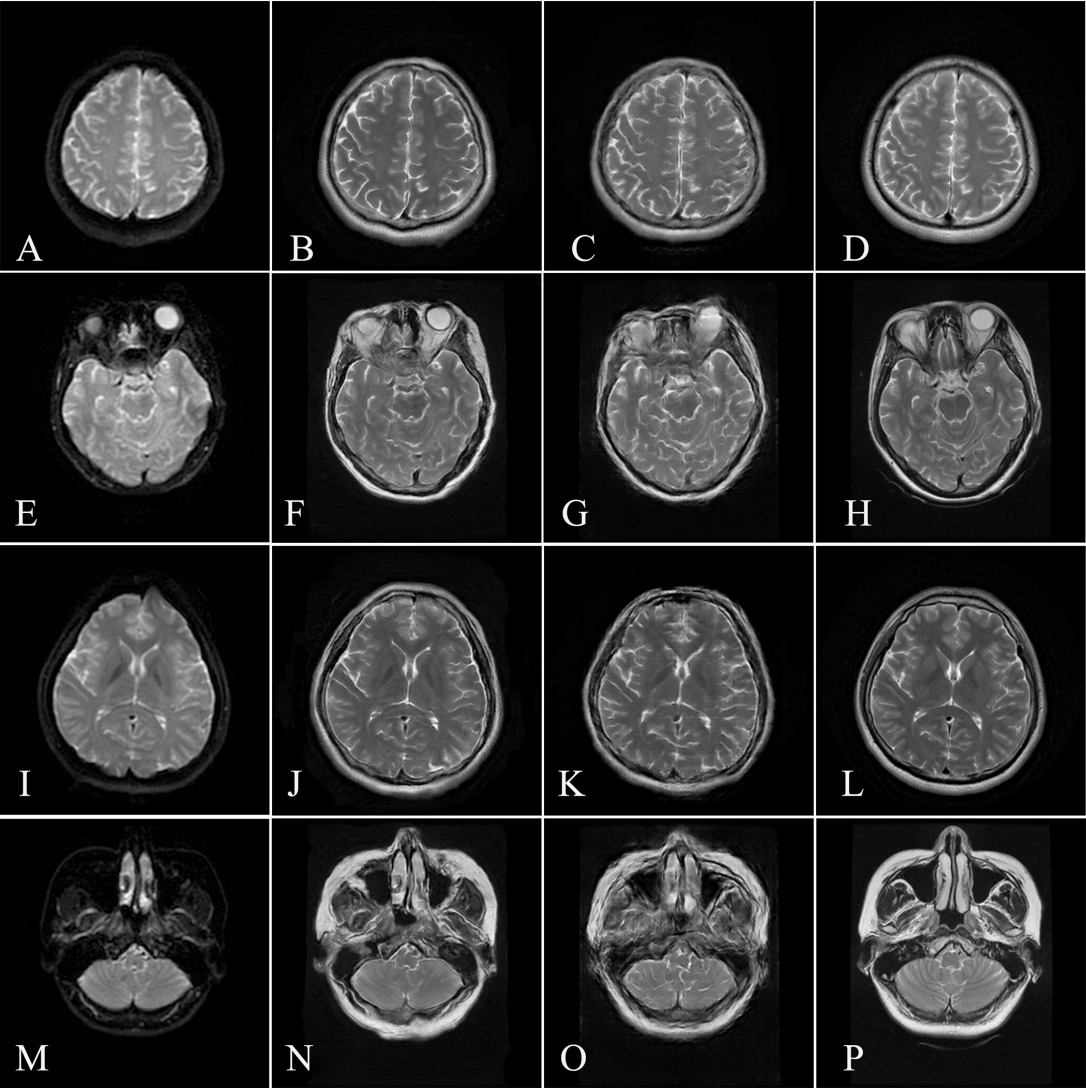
Representative image in the test set. The left column shows the DWI b0 image (A/E/I/M), the middle column shows the sT2WI images generated by Hi-Net (B/F/J/N), and pix2pix (C/G/K/O) the respectively, and the right column shows the original T2WI images (D/H/L/P).
